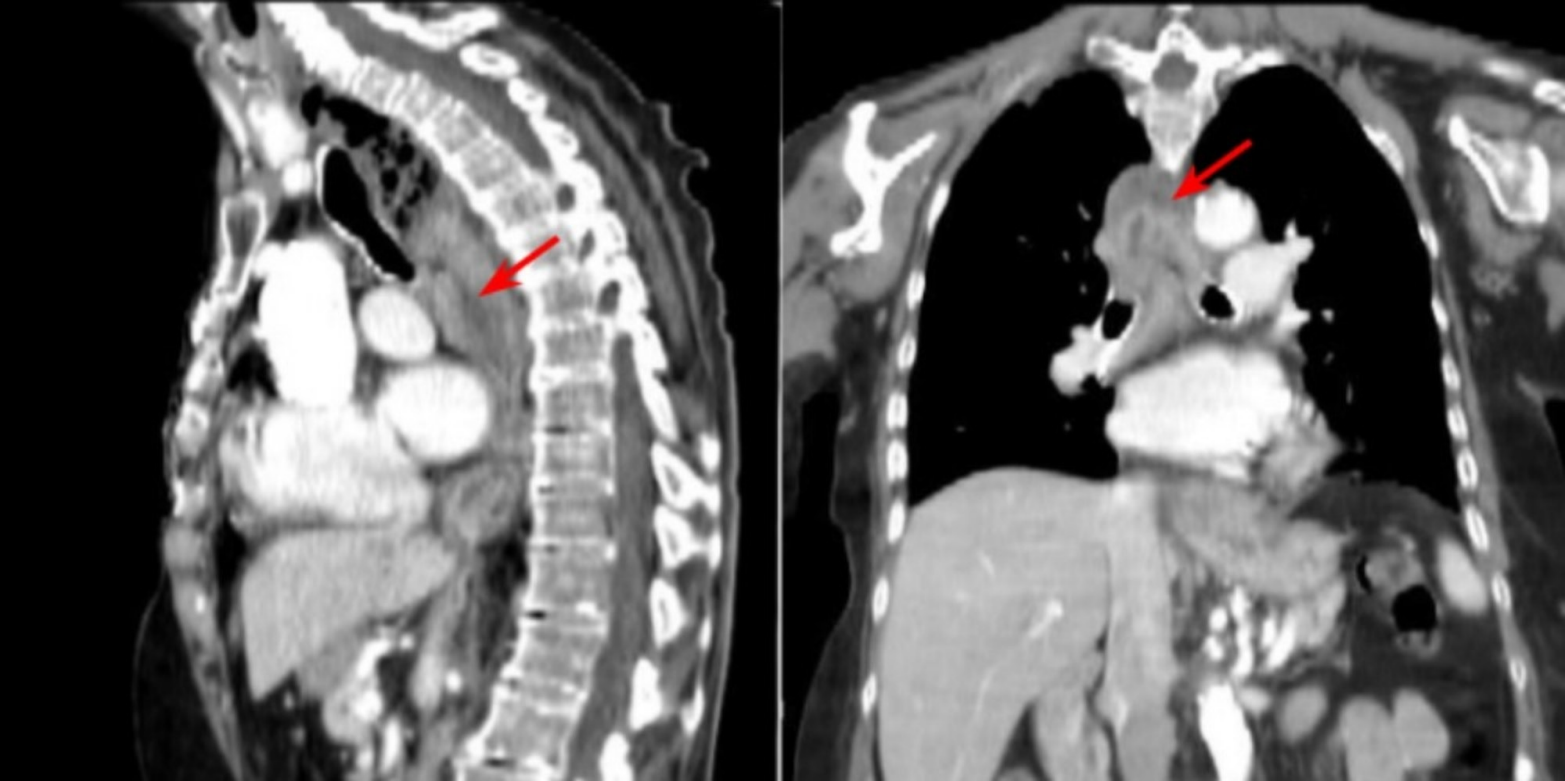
Computed tomography (CT) of the chest with contrast demonstrating marked esophageal dilatation with fluid within the entire length of the esophagus
